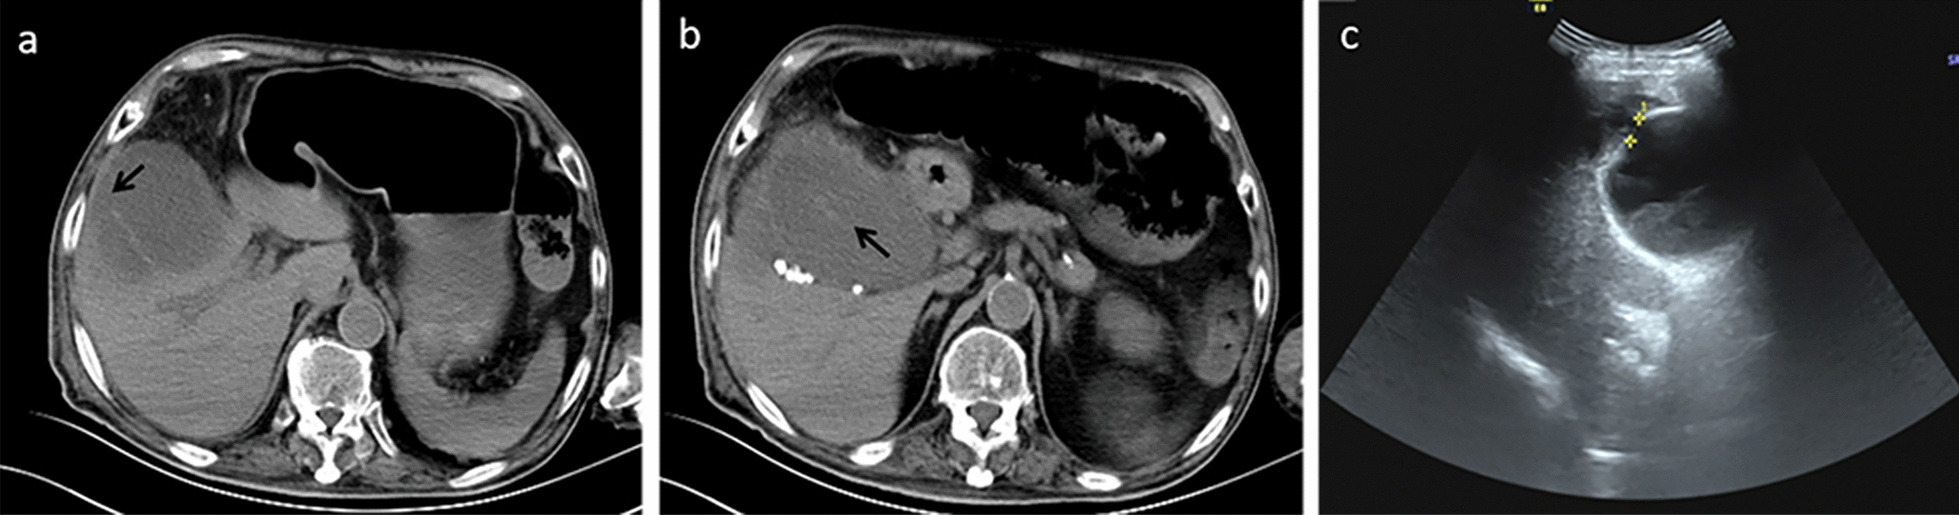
An 86-year-old male with defects in the gallbladder wall. a Nonenhanced CT showed defects in the gallbladder wall (arrow). b Nonenhanced CT showed a sloughed intraluminal membrane (arrow). c US showed defects in the gallbladder wall and a subhepatic hypoechoic area
